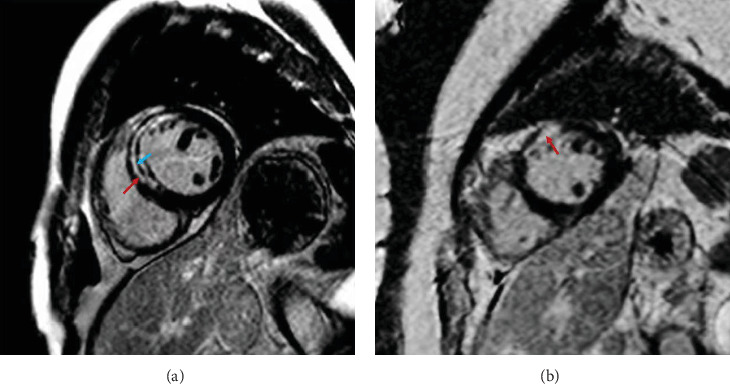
Delayed-enhancement cardiac MRI findings in two patients with acute myocardial infarction caused by occlusion of the left anterior descending coronary artery. (a) MVO in a patient without Shenfu. (b) Another AMI patient treated with Shenfu for 5 days. Only focal endocardial LGE was found. Red arrow: myocardial infarction area. Blue arrow: MVO area of myocardial infarction.
